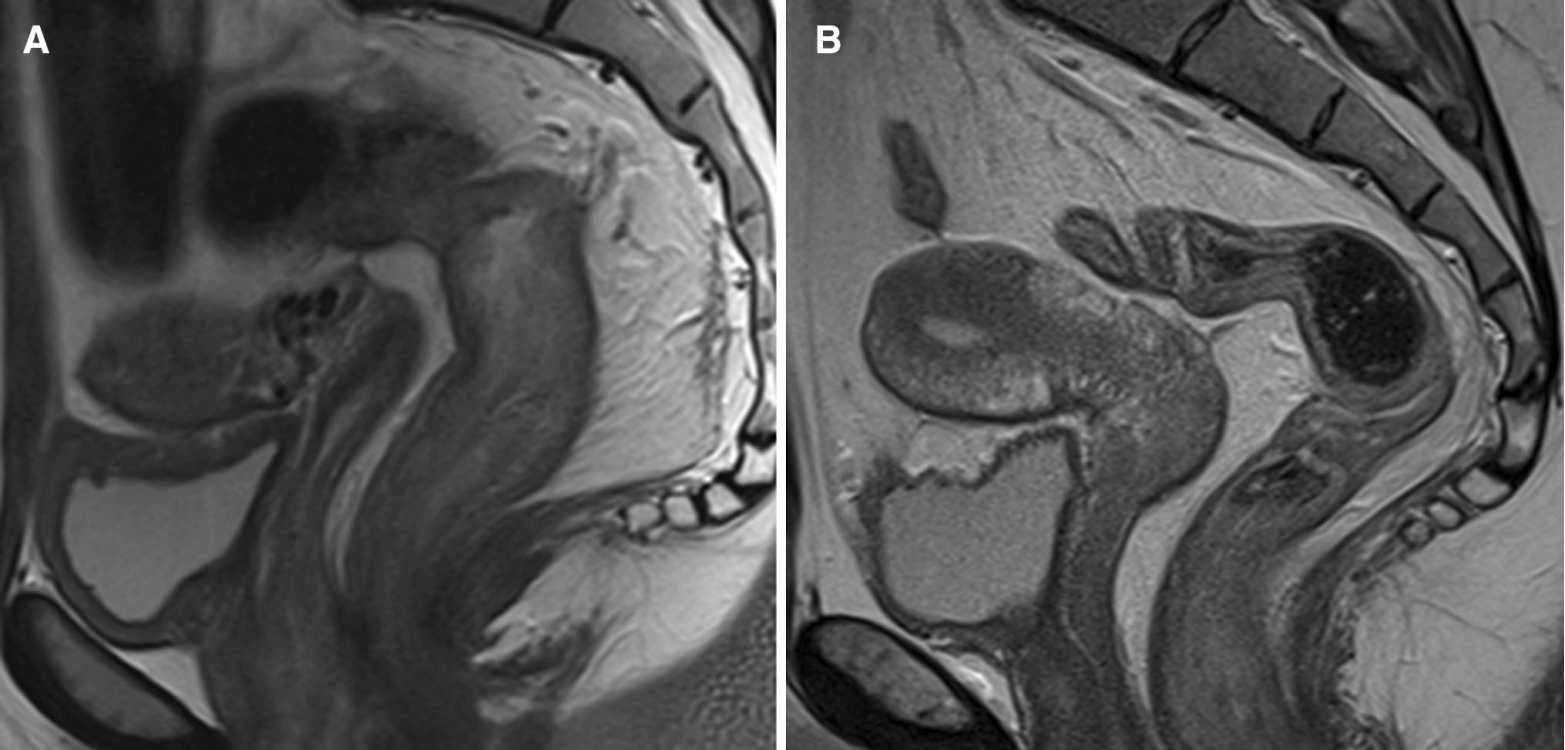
Sagittal T2-weighted image of two different patients with Crohn’s disease. A A 25-year-old female with ulcerative proctitis at endoscopy. The image shows increased amount of mesorectal fat tissue (creeping fat) and a subtle increase of perimural vascularity (‘comb sign’) in addition to rectal wall thickening. B A 24-year-old female with no signs of proctitis at endoscopy. There is no increased amount of mesorectal fat tissue and the rectum shows no abnormal MRI features
